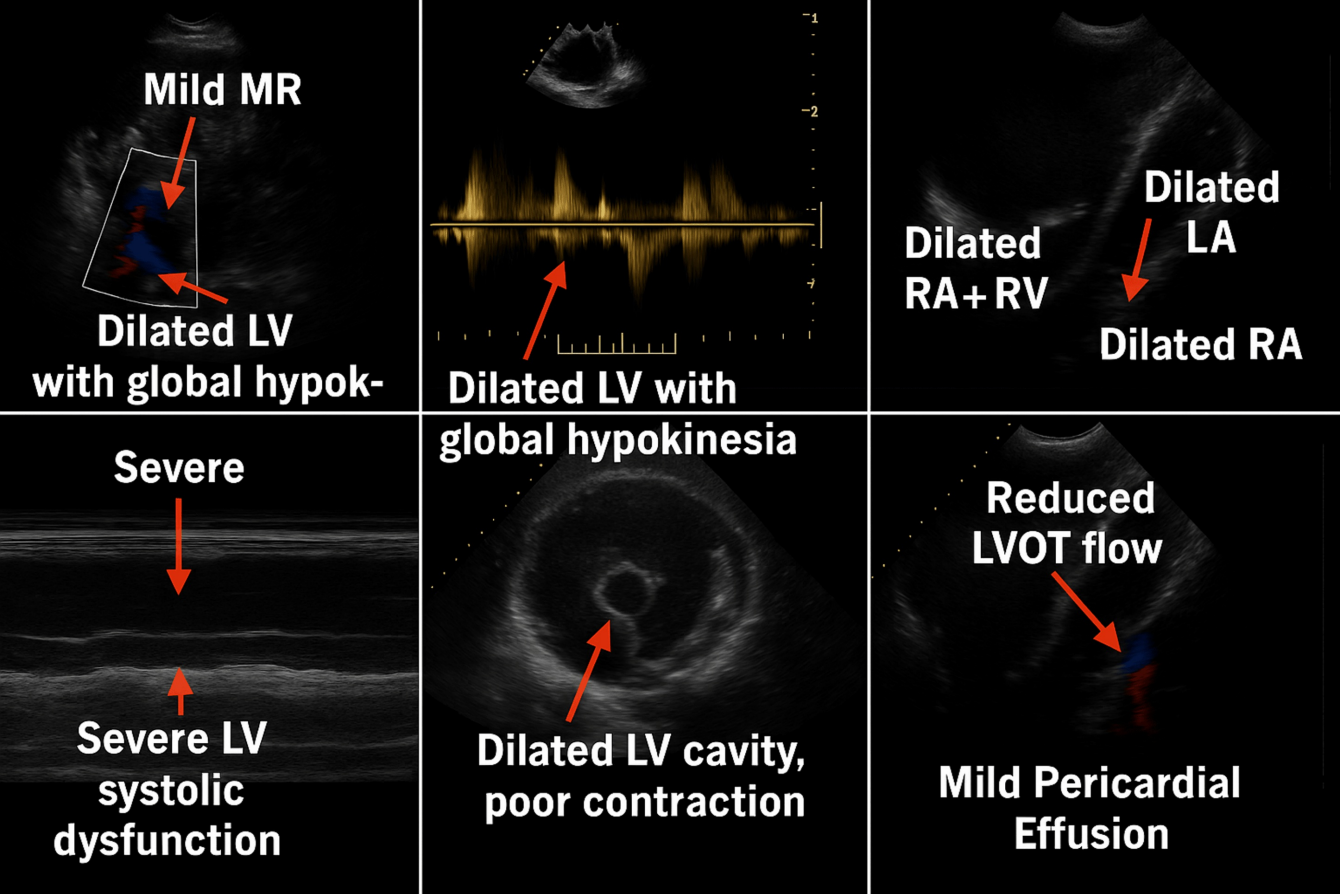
Echocardiography image of case 1 LVOT: Left ventricular outflow tract
